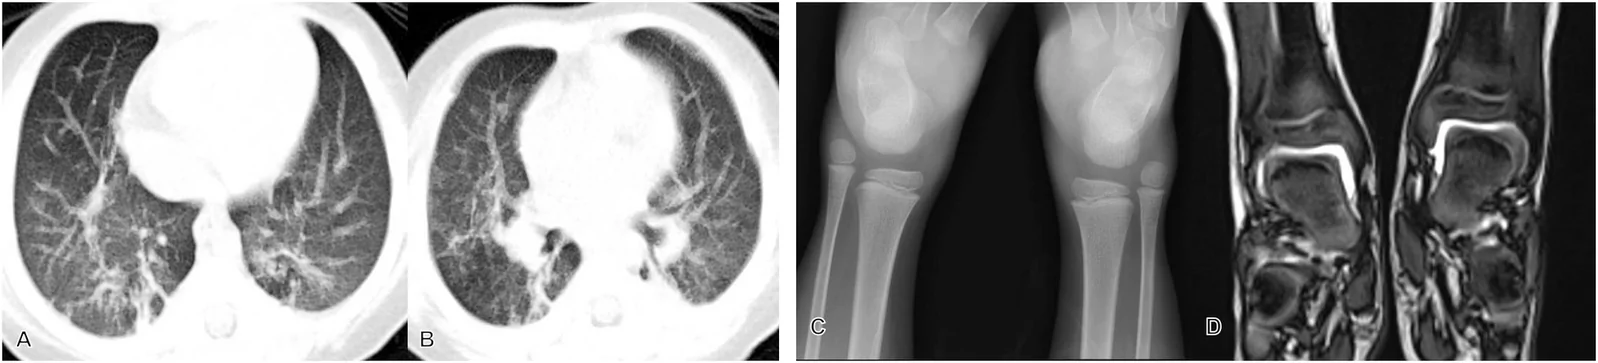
Imaging findings in patients 1 and 5. (A,B) Chest computed tomography (CT) images of patient 1 demonstrating bilateral pulmonary involvement with multiple parenchymal and interstitial lesions. (C) Plain radiographs of the ankles in patient 5 showing bilateral soft tissue swelling, with preserved joint surfaces and normal joint alignment. (D) Magnetic resonance imaging (MRI) of the ankles in Patient 5 revealing bilateral joint effusion and marked synovial thickening with contrast enhancement.
